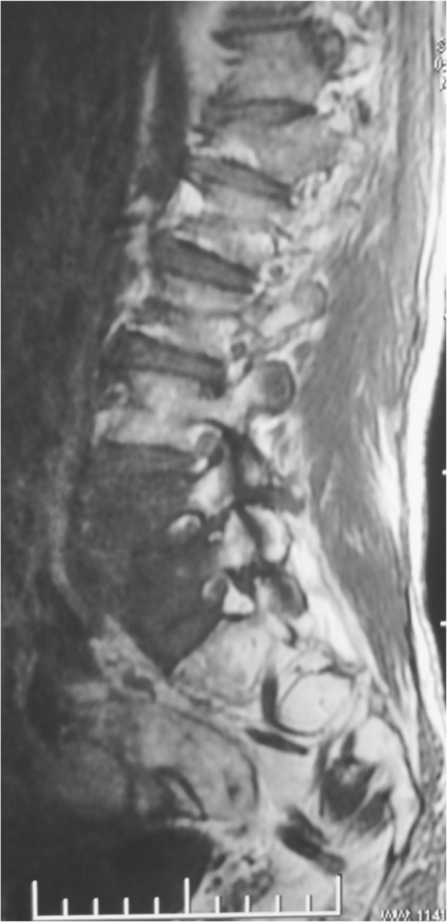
Preoperative magnetic resonance image of a 43 year old male patient with lumbosacral tuberculosis
